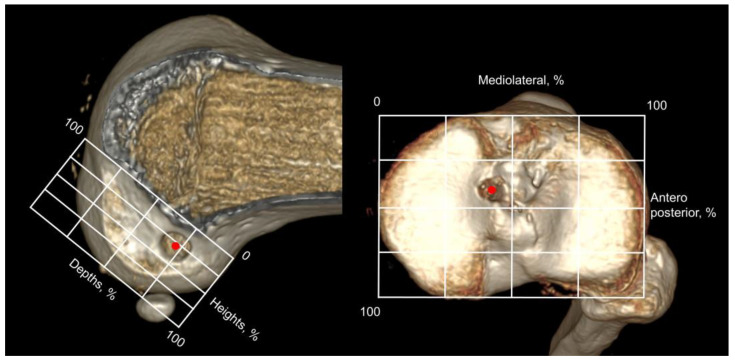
The center of the femoral and tibial tunnel (red dots) was calculated on 3-dimensional CT with the quadrant method. The height and depths of femoral tunnel position and anteroposterior and mediolateral tibial tunnel position were measured.
